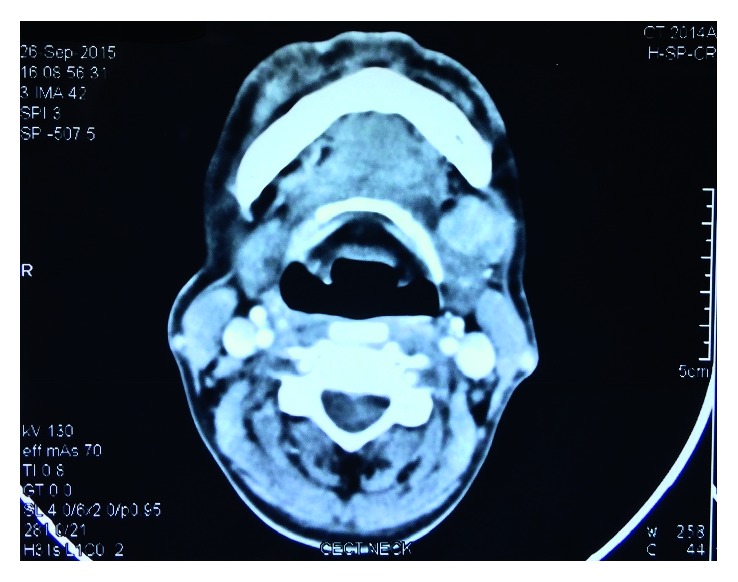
Contrast-enhanced computed tomography. CECT axial section shows ill-defined heterogeneously enhancing soft tissue lesion in the left submandibular region with no obvious bone erosion. Few subcentimetric nodes are seen along bilateral submandibular and upper jugular chains.
